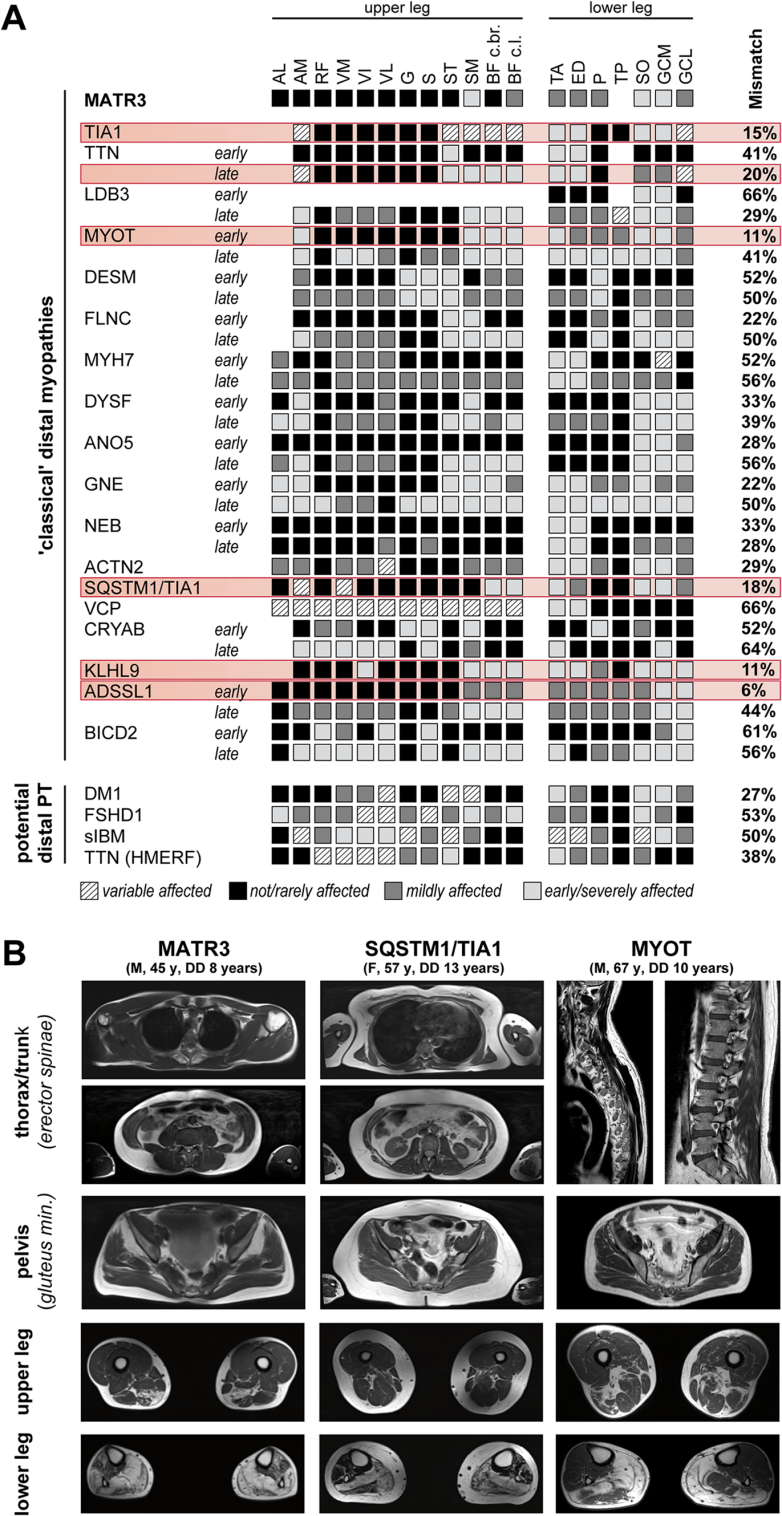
Comparison of the established MATR3-pattern to other distal myopathies. a Literature-based pattern of different distal myopathies and the respective mismatch to the established MATR3-pattern. Both ‘classical’ distal myopathies (obligatory distal phenotype), as well as myopathies with comparably high prevalence and potential predominant distal phenotype (potential distal PT) are included. Disease entities showing relevant similarities (mismatch < 20%) are highlighted in red. b Sample MRI findings in patients with distal myopathies determined to show similar patterns in lower limb muscular affection compared to MATR3-myopathy. Gluteal and paraspinal musculature is spared in patients with MYOT- and SQSTM1/TIA1-associated distal myopathy. *DD* disease duration, *y* years, *PT* phenotype, *F* female, *M* male, *FSHD* Facioscapulohumeral muscular dystrophy 1, *DM1* Myotonic dystrophy 1, *sIBM* sporadic Inclusion body myositis, *HMERF* Hereditary myopathy with early respiratory failure, *AL* adductor longum, *AM* adductor magnus, *RF* rectus femoris, *VM* vastus medialis, *VI* vastus intermedius, *VL* vastus lateralis, *G* gracilis, *S* sartorius, *ST* semitendinosus, *SM* semimembranosus, *BF c.br.* biceps femoris short head (caput breve), *BF c.l.* biceps femoris long head (caput longum), *TA* tibialis anterior, *ED* extensor digitorum, *P* popliteus, *TP* tibialis posterior, *SO* soleus, *GCM* gastrocnemius medialis, *GCL* gastrocnemius lateralis, *aff.* affected
